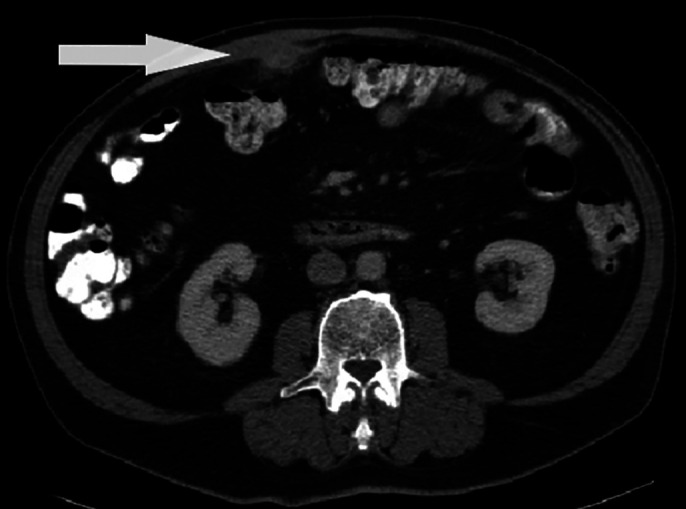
Initial abdominal and pelvic computed tomography with contrast showing an ill-defined round density mass (white arrow) measuring 2.5 cm in greatest diameter adherent to or arising from the posterior margin of the abdominal wall.
